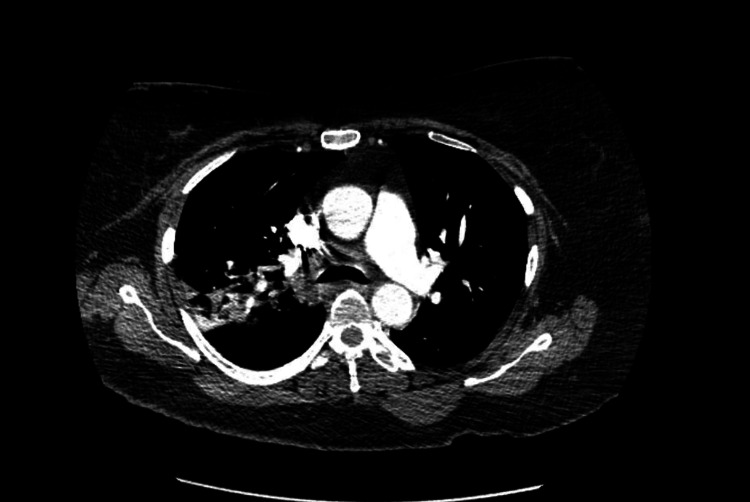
CTA chest The findings of the CTA chest are as follows: Pulmonary arteries are well opacified. There is no evidence of pulmonary thromboembolism. The thoracic aorta is well opacified. There is no evidence of aneurysm or dissection. No mediastinal or hilar adenopathy of significance. Heart size is borderline enlarged and there is no pericardial effusion. Also, there is minimal coronary artery calcification. There is significant airspace disease in the night upper lobe consistent with pneumonia. There is also pulmonary opacity in the right lower lobe also suspicious for pneumonia. The left lung is grossly clear other than some minimal atelectasis. No pleural effusion in either lung. Imaging obtained through the upper abdomen does not demonstrate any acute finding. There was no acute osseous abnormality. CTA: Computed tomography angiography
